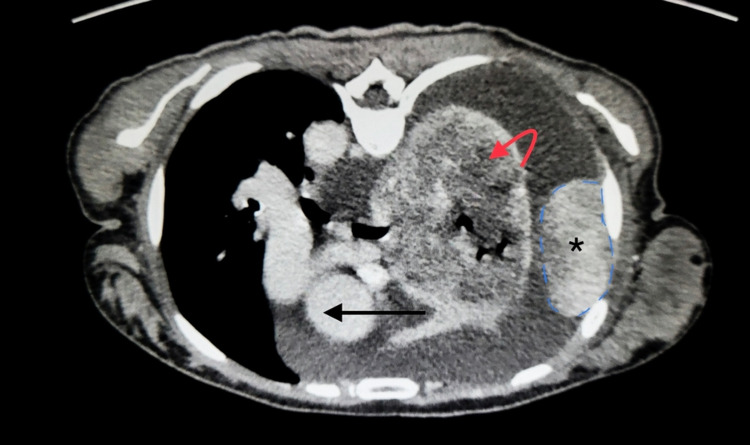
Computed Tomography (CT) of chest with contrast (axial view) showing 6.8x4.1x6.4 cm pleural mass (*) highlighted with blue dashes, with large pleural effusion causing compression atelectasis (red arrow) and mediastinal shift to left (black arrow).
